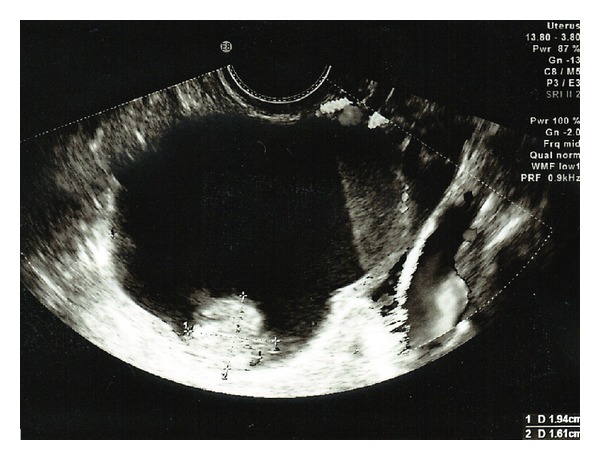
Pelvic ultrasound, showing the large complex ovarian cyst with layered low level echoes measuring 1.9 × 1.6 cm.
